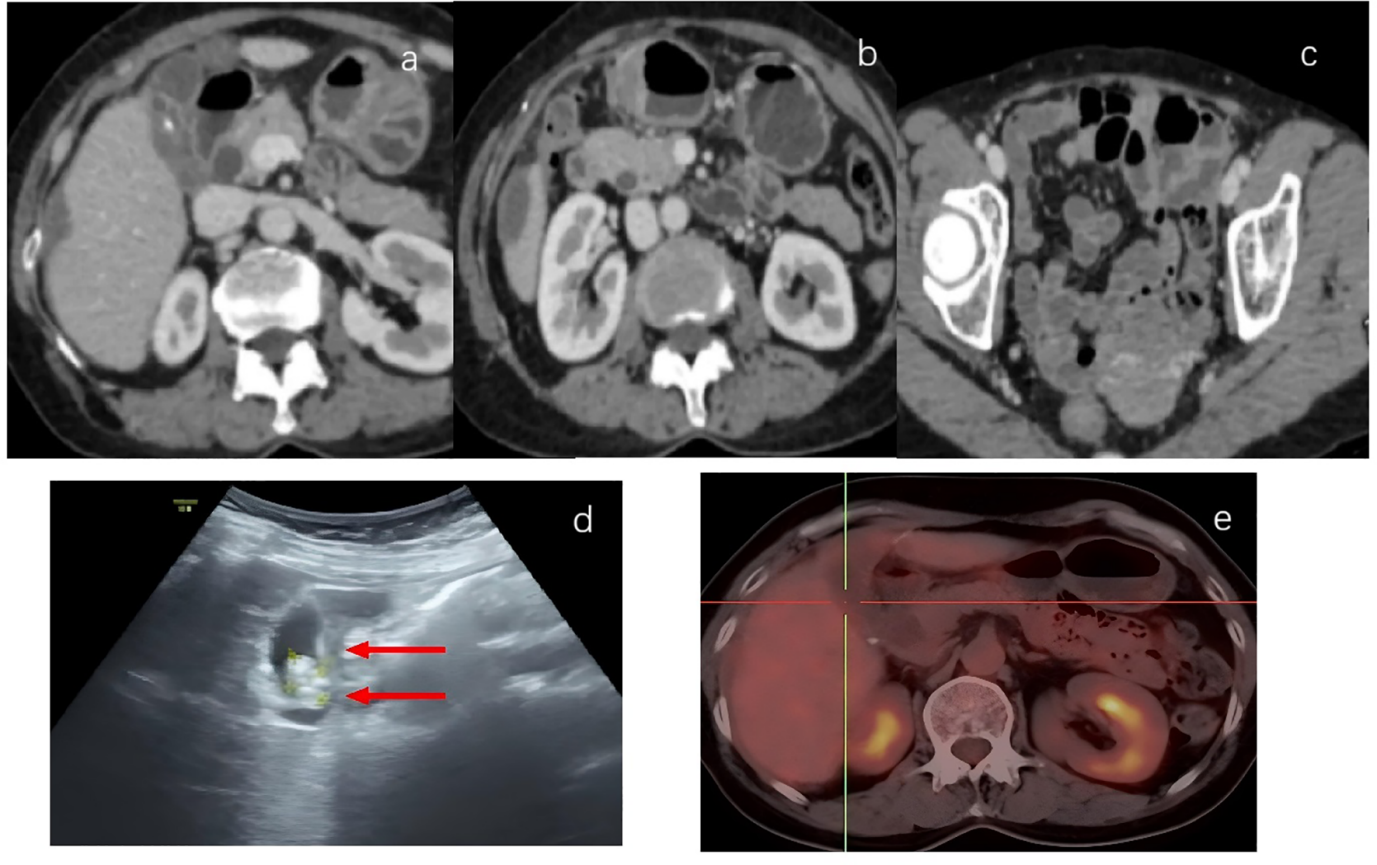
Post-conversion imaging: (a–c) Contrast-enhanced abdominal CT images: After conversion therapy figures (a–c) show post-treatment changes of gallbladder cancer, with mild gallbladder wall thickening and ascites resolution. (d) Ultrasound images: The gallbladder size was normal, the wall was thickened (red arrow), and two polypoid changes were visible in the cavity. (e) PET-CT images: the gallbladder showed hypermetabolism with a maximum SUV uptake of 2.9, which is within the normal range of gallbladder function.
